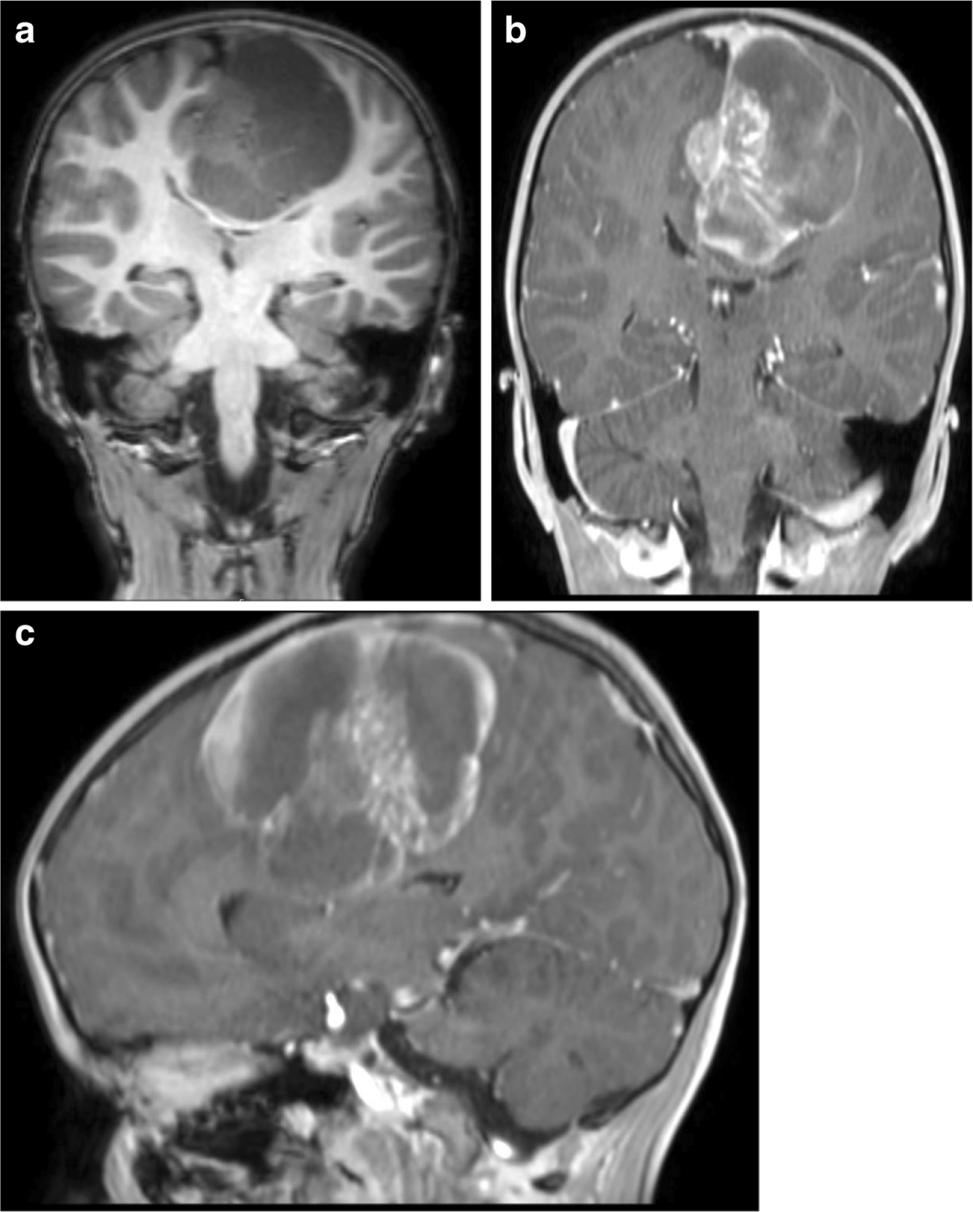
a Images from Case 3 of a 5-year-old male presenting with a left parietal lobe tumor with transfalcine extension. Coronal pre-contrast T1-weighted MRI. b Coronal post-contrast T1-weighted MRI. c Sagittal post-contrast T1-weighted MRI
